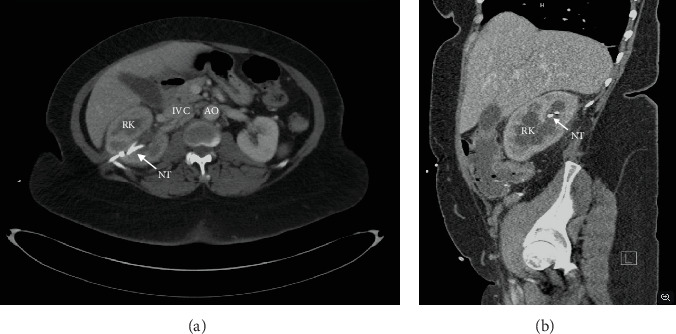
(a) Donor evaluation axial abdomen/pelvis CT with contrast depicting moderate right hydroureteronephrosis of the right kidney. (b) Sagittal CT abdomen/pelvis with contrast depicting moderate right hydroureteronephrosis with a transition point in the right mid ureter, compatible with stricture. The right percutaneous nephrostomy tube (NT) is able to be visualized in both planes, terminating within a right upper pole calyx. There is a 1.3 cm indeterminate hypodense lesion in the left upper pole, stable in size compared to the prior CT on 02/16/2023. IVC, inferior vena cava; AO, aorta; RK, right kidney; NT, nephrostomy tube.
